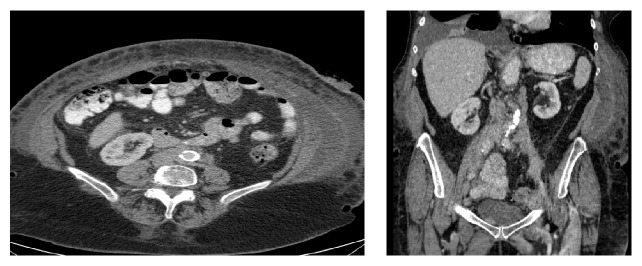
Coronal and transverse CT imaging of para-aortic lymphadenopathy.
